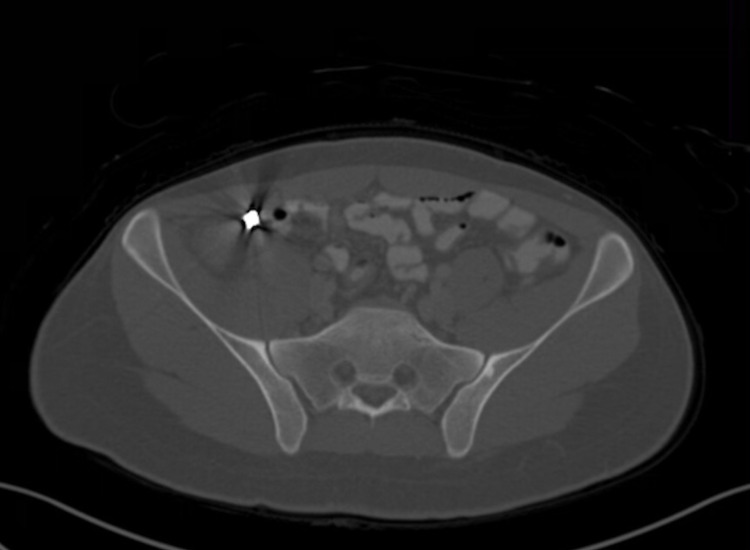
Bone Window of CT Scan Shows the entirety of the metallic foreign body causing streak artifact.
